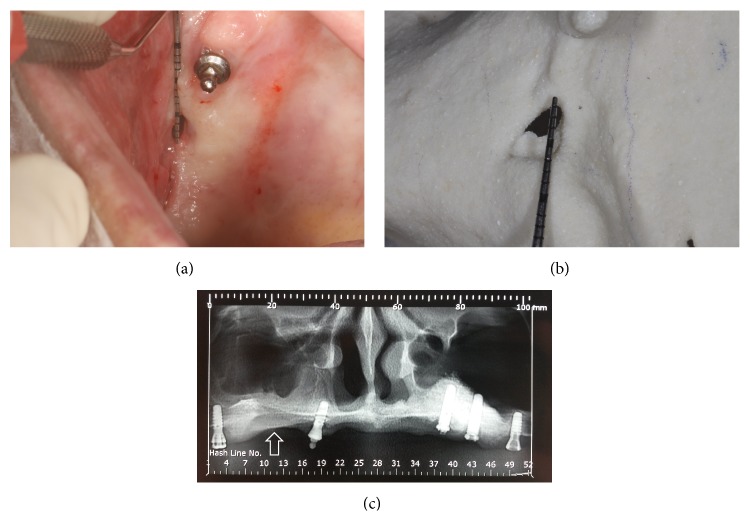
Initial appearance of the patient's condition upon (a) inspection and (b) prototyping, the arrow indicates an 5 mm opening, and (c) radiographic appearance, the arrow indicates oroantral communication.
